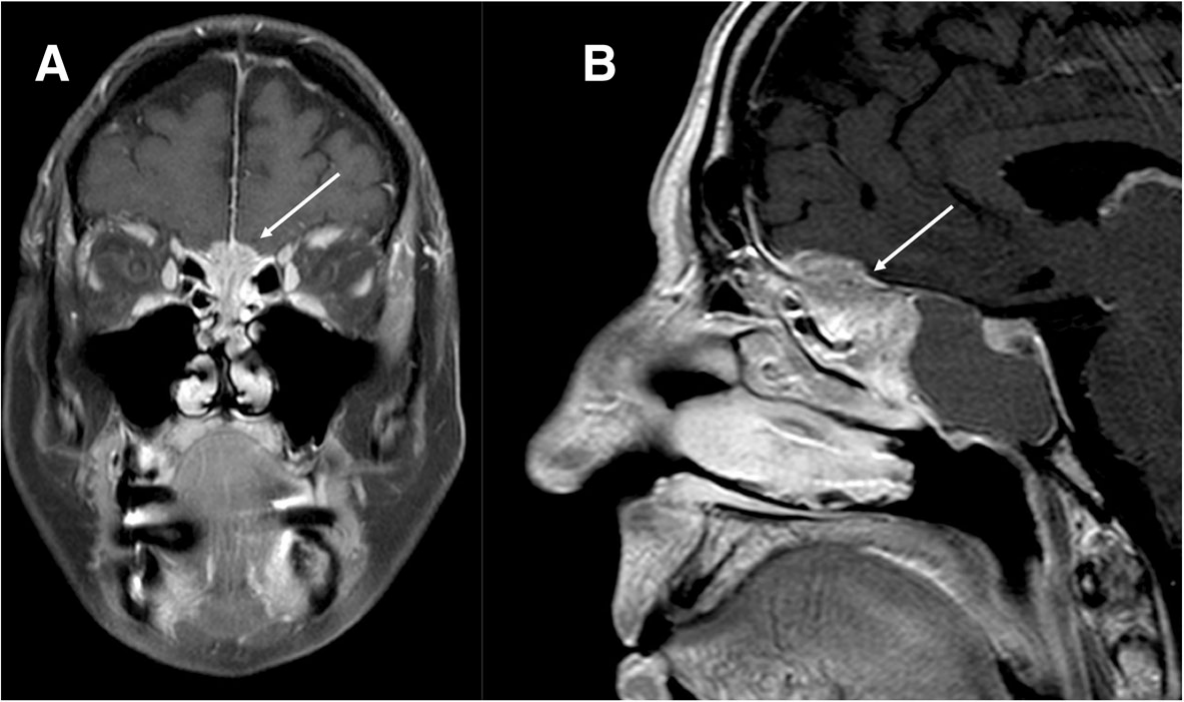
Esthesioneuroblastoma. MRI T1-weighted fat-suppressed post-gadolinium sequence, coronal (a) and sagittal (b) images show a marked enhanced large median mass (arrows) in the sinonasal region growing superiorly into the inferior frontal region of the brain
